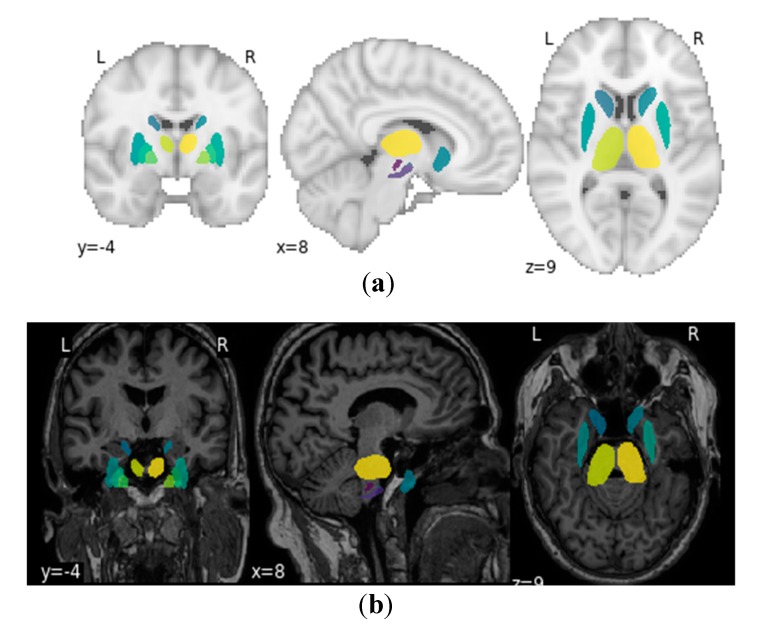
ROI plotting on (a) MNI-ICBM-152 template and (b) an acquired MRI scan from PPMI.
